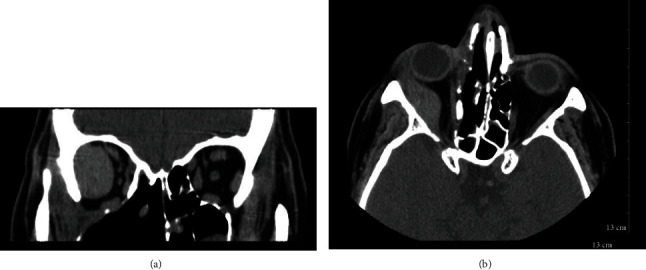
Coronal (a) and axial (b) CT scans showing large orbital mass with lateral rectus involvement.
